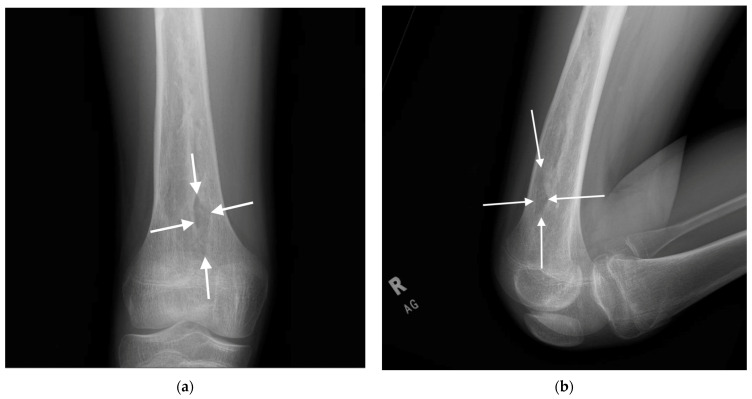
Radiographs of the right anterior distal femoral diaphysis taken seven months postoperatively. White arrows denote the outer margins of the cortical perforation. (a) Anteroposterior (AP) radiographs. (b) Lateral radiographs.
